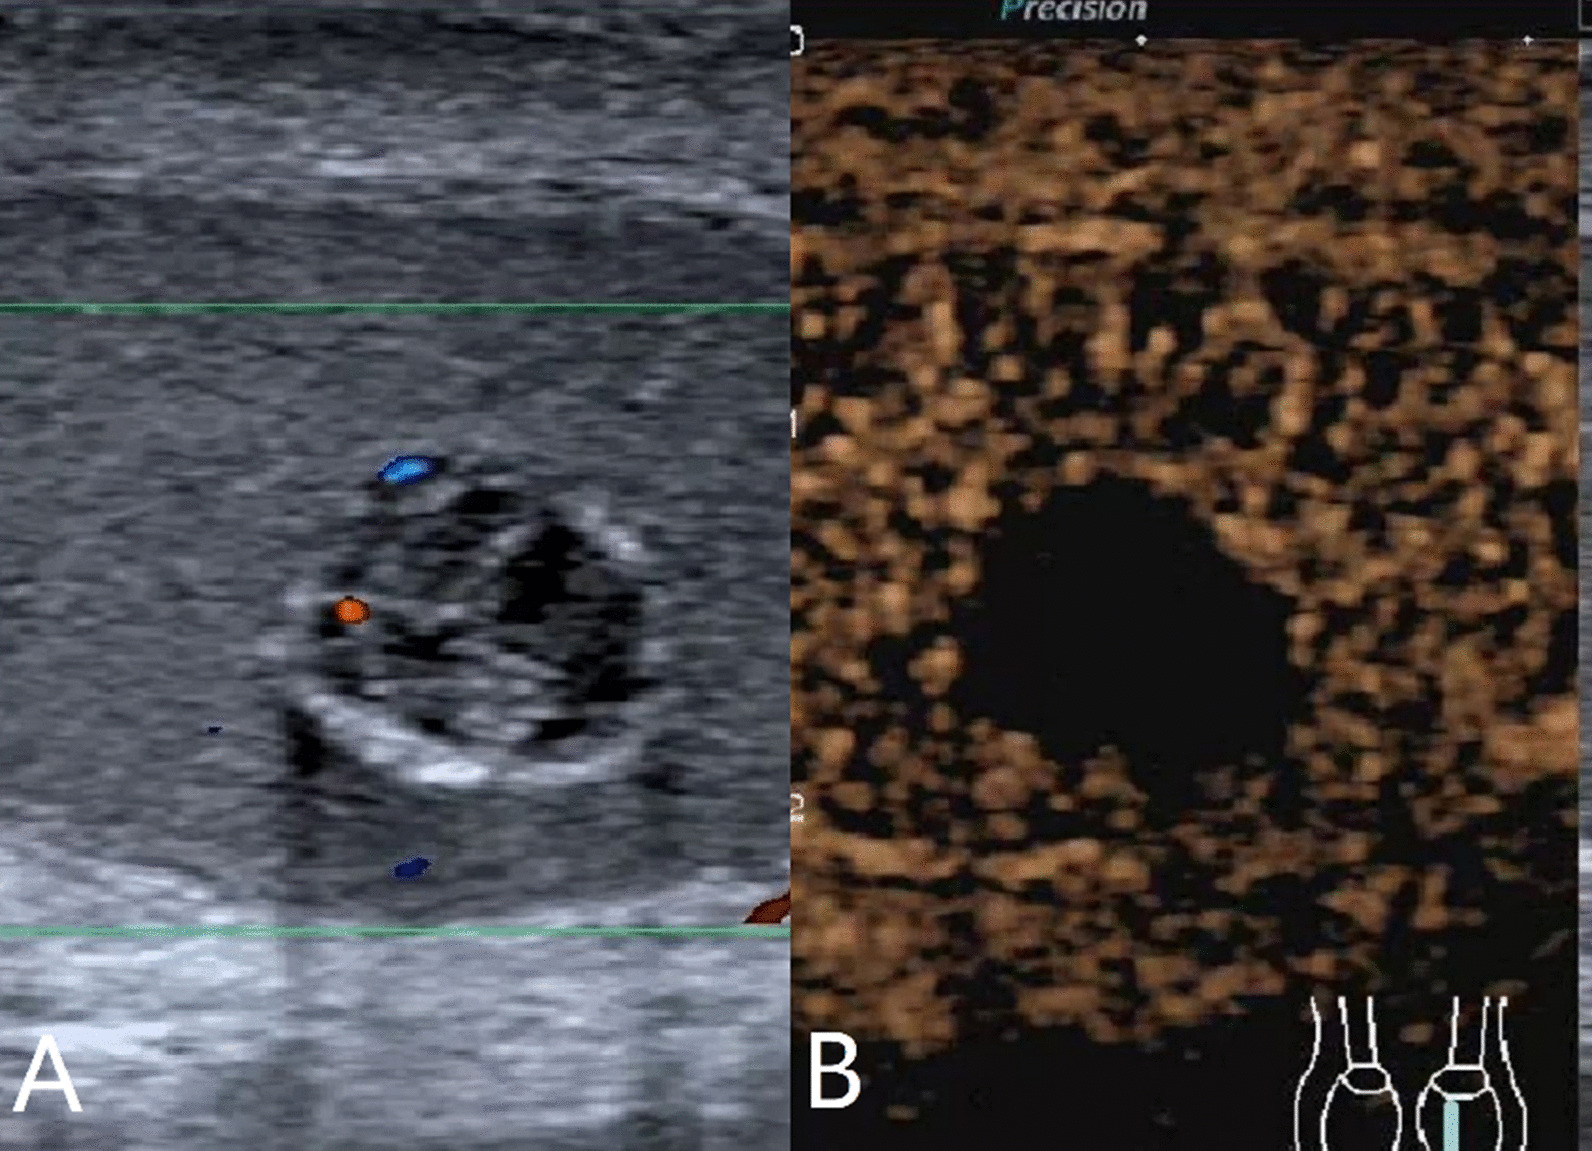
Testicular epidermoid cyst. A Conventional ultrasound showed an uneven echo in the left testis, calcification in the periphery and blood flow inside. This was considered to be a malignant tumor. B CEUS showed no enhancement; the diagnosis was epidermoid cyst
